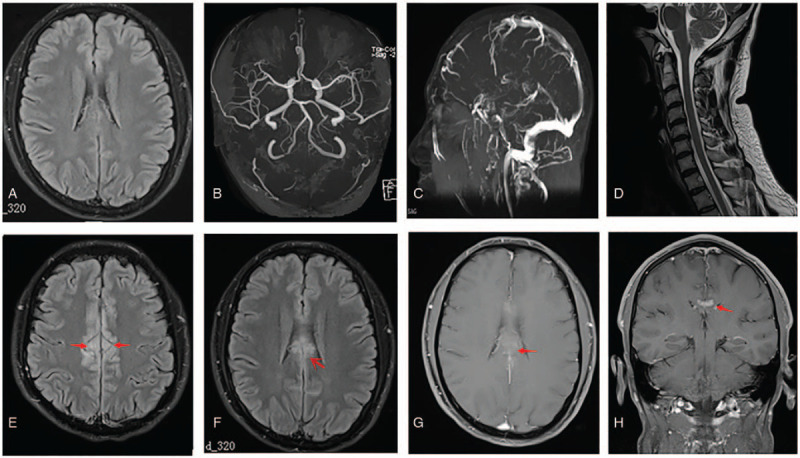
Magnetic resonance (MR) images of the brain and cervical spine. An initial fluid-attenuated inversion recovery (FLAIR) image (A), brain MR angiography (B), brain MR venography (C), and cervical spine MR images (D). All show no obvious abnormalities. Repeated FLAIR image after the condition worsened, demonstrating an abnormal hyperintense signal in the medial aspect of the bilateral frontal lobes and involvement of the cingulate cortex (E and F, arrows); a gadolinium-enhanced T1-weighted image showing enhancement in the parts above the lesions (G, arrow); and abnormal enhanced meninges (H, arrow).
